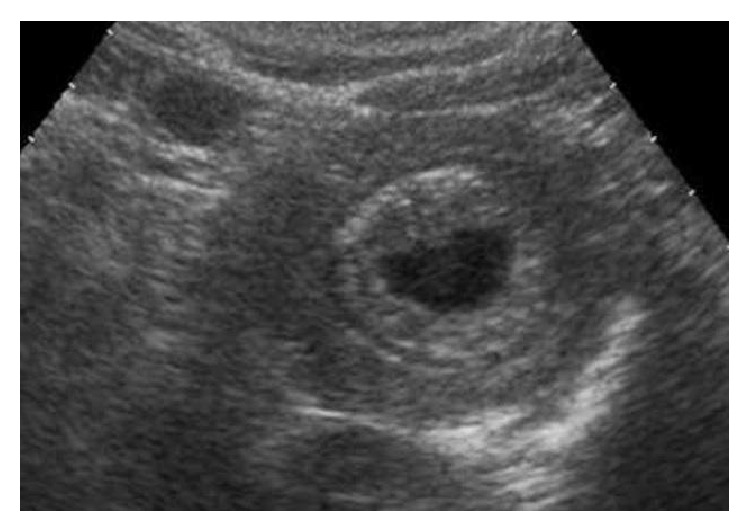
Abdominal ultrasound image depicts the typical image of intussusception with a simple cyst inside.
